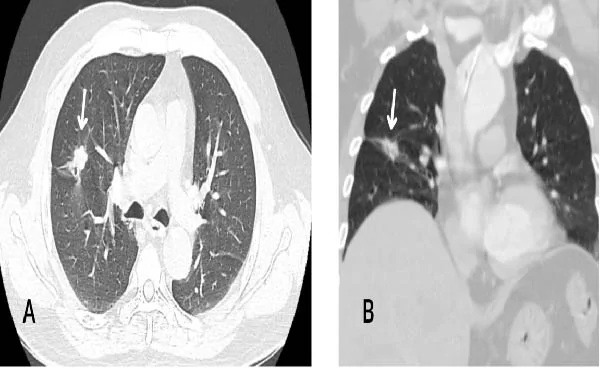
Axial (A) and coronal (B) CT images of the chest demonstrate a 2.1 cm spiculated pulmonary nodule located in the lateral segment (S4) of the right middle lobe (arrow). The lesion exhibits irregular margins with radiating spicules, raising suspicion for a malignant etiology.
